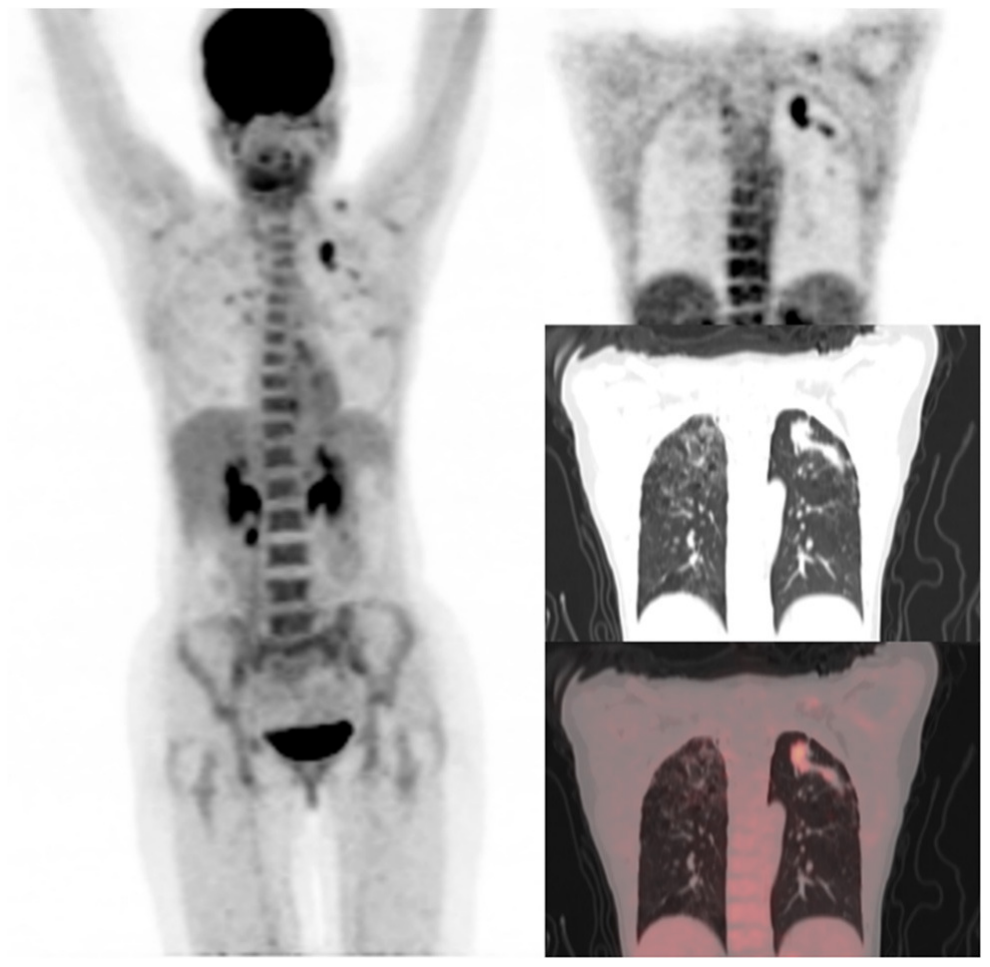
A 31-year-old HIV-infected female who completed a six-month course of anti-tuberculous medication for drug-sensitive pulmonary tuberculosis. Maximum intensity projection scan (MIPS) on the left shows a focal area of residual metabolic activity in the left upper lobe. This focus of residual metabolic activity is confirmed to be in left upper lobe lung nodules on the coronal section of PET, CT, and fused PET/CT images shown on the right. Fibrocavitory changes seen in the lungs bilaterally do not demonstrate significant residual metabolic activity.
